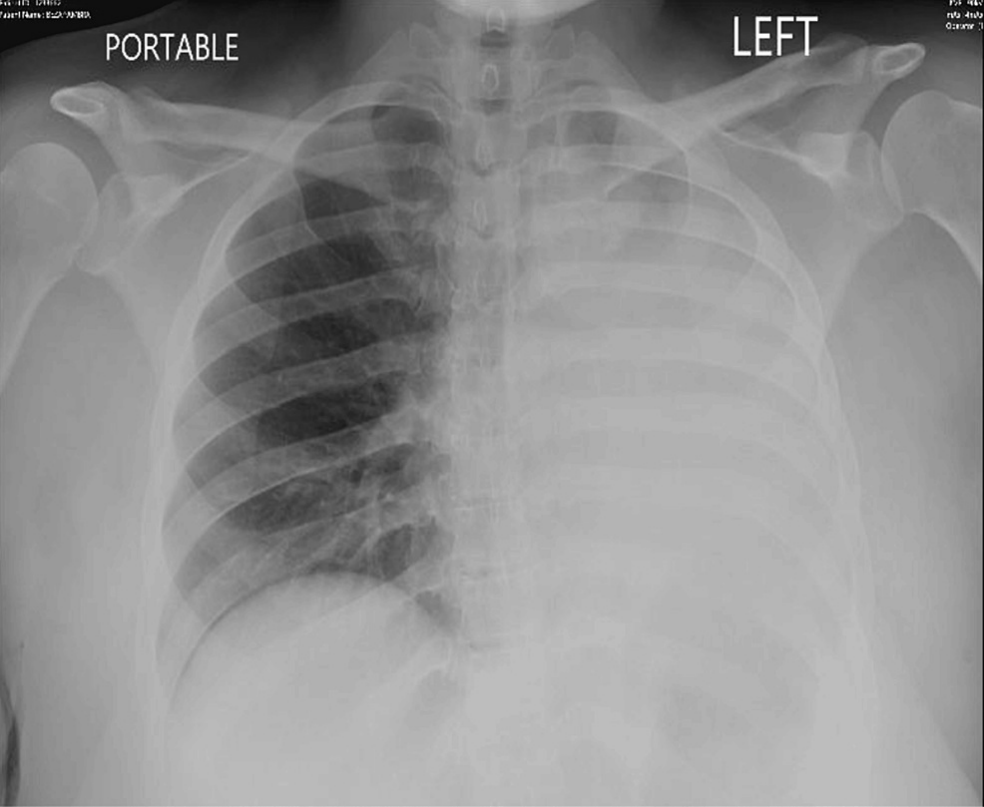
Chest X-ray on admission, prior to bronchoscopy
